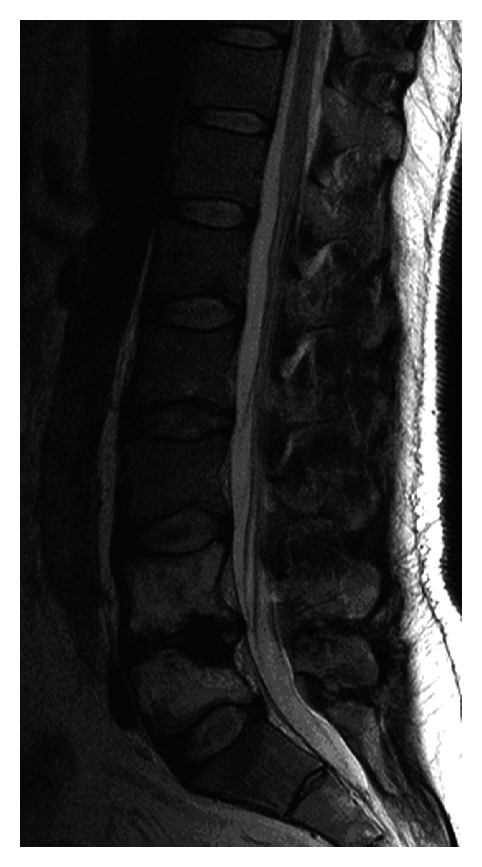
A follow-up magnetic resonance imaging (MRI) examination of the spine performed in September 2008 showed high signal intensity of L4 and L5 lumbar vertebral bodies in T2-weighted images (WIs) as signs of healing, with loss of height from infective destruction. There was no spinal canal stenosis.
